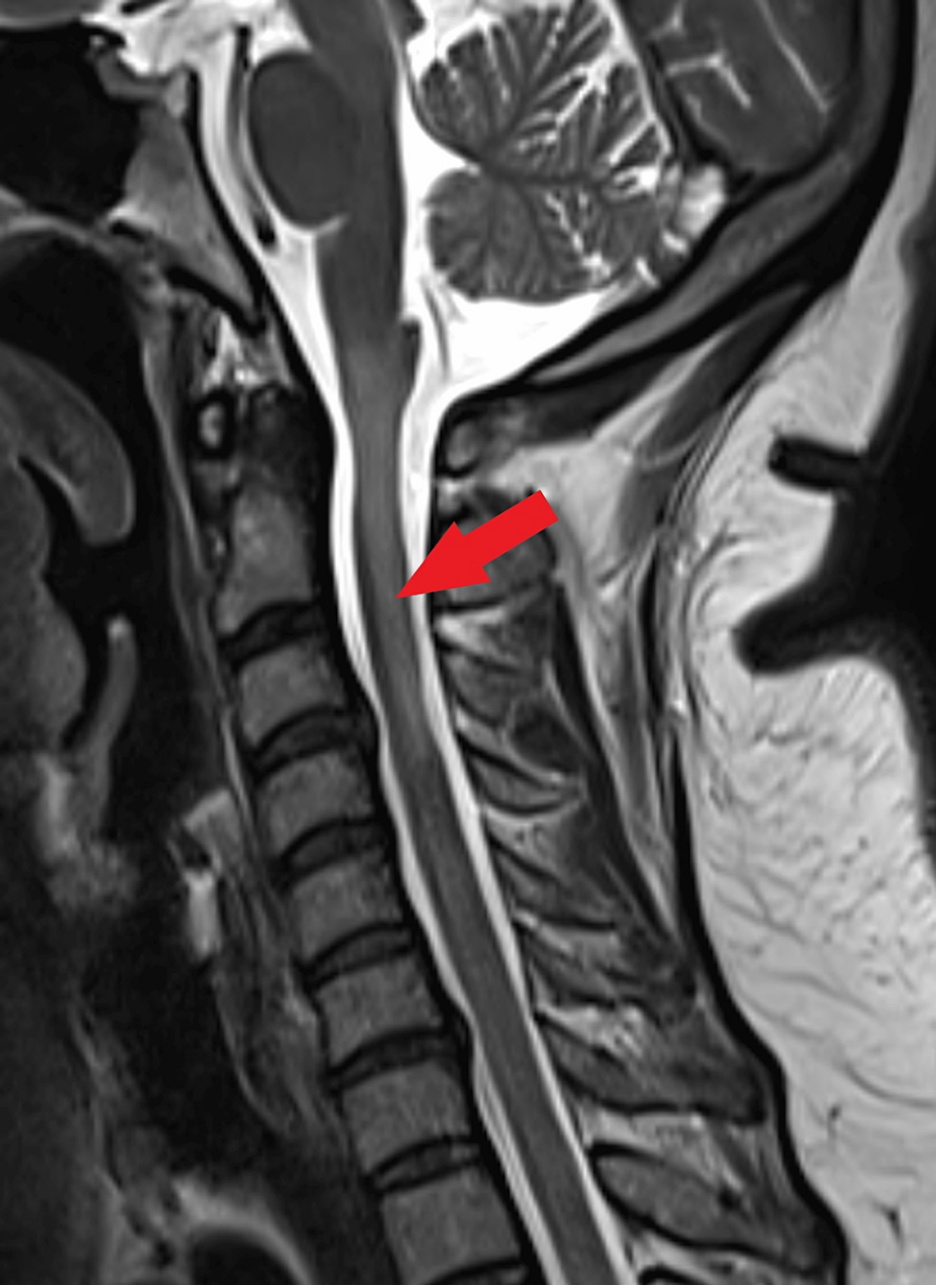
Long segment hyperintense signal in the cervical cord extending from the cervicomedullary junction to upper end of C4 vertebral body on T2-weighted sagittal image.
